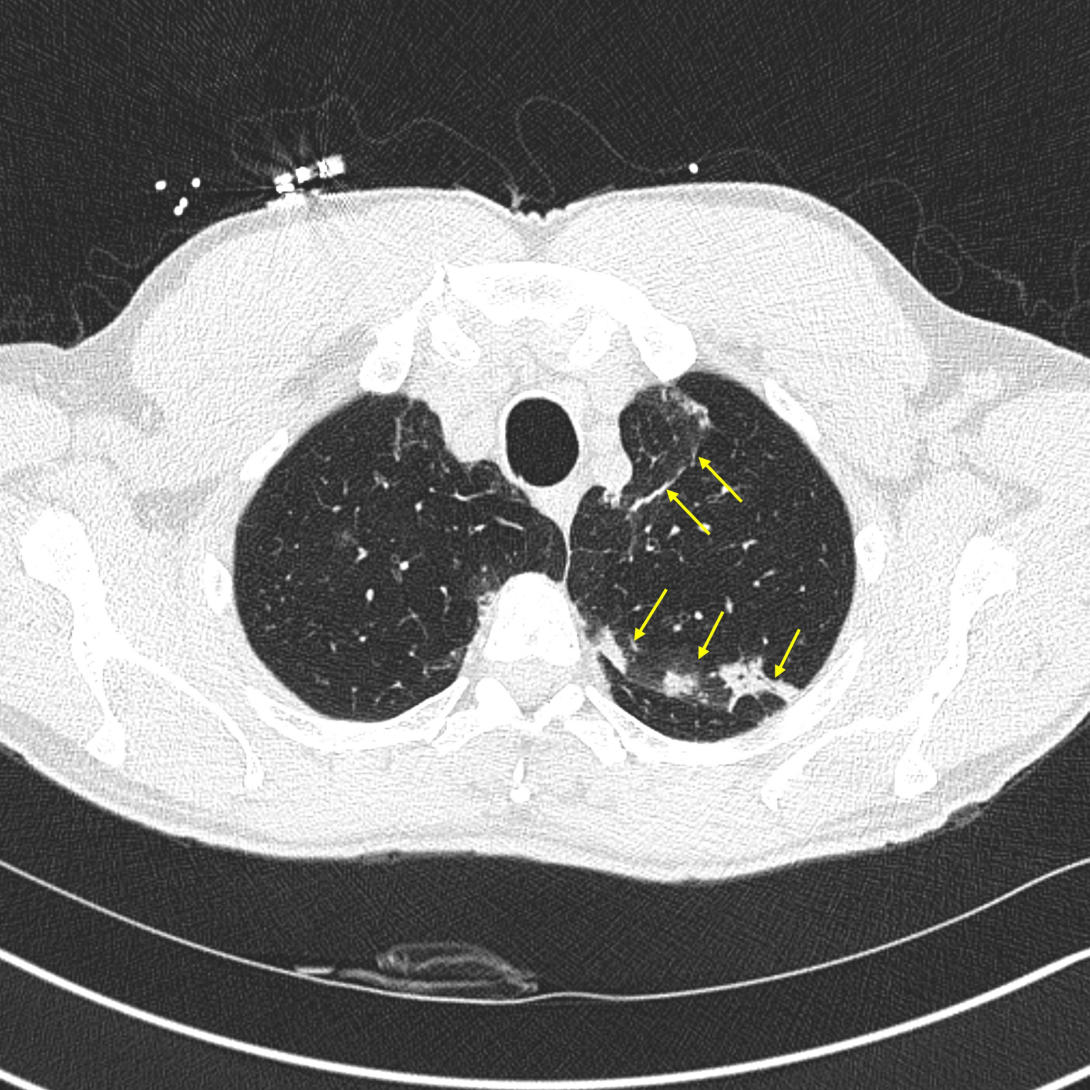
Computed tomography of the chest revealing ground-glass opacities (arrows).
